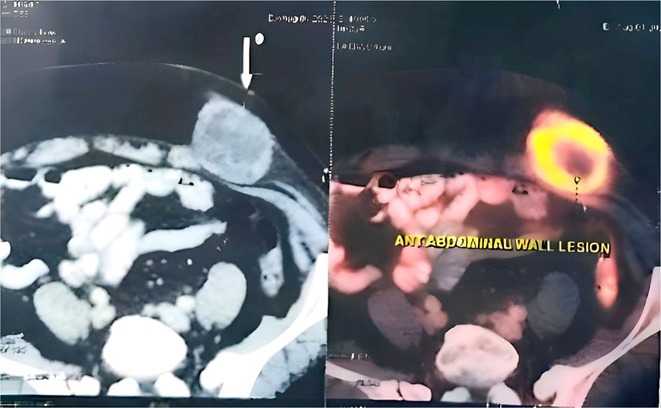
FIGURE 1 CECT abdomen showing well‐defined oval hypodense lesion of size 5.3 × 5.2 × 4.2 cm seen in deep subcutaneous plane of anterior abdominal wall with maintained fat plane. (b) FDG avid heterogeneously enhancing soft tissue mass with central necrosis.
